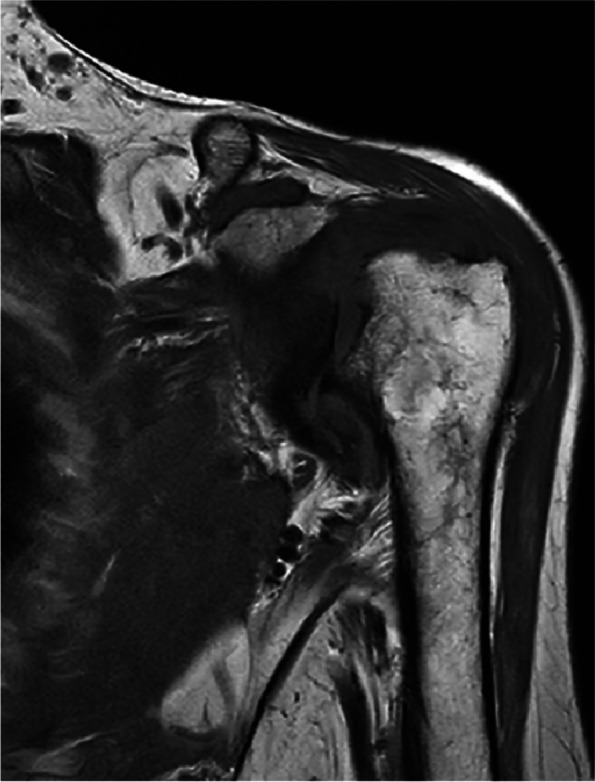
Coronal MRI of the left shoulder joint
